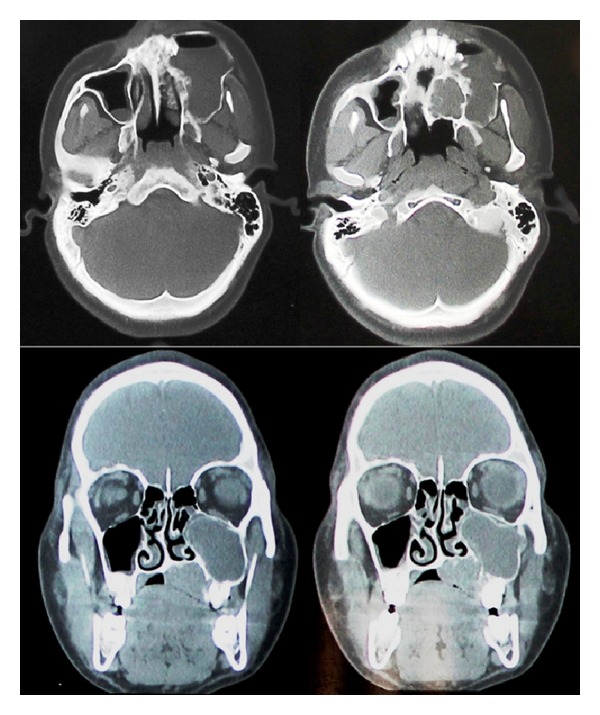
Axial and coronal CT views showing large osteolytic lesion/s involving left maxilla with continuity of buccal and palatal lesions.
